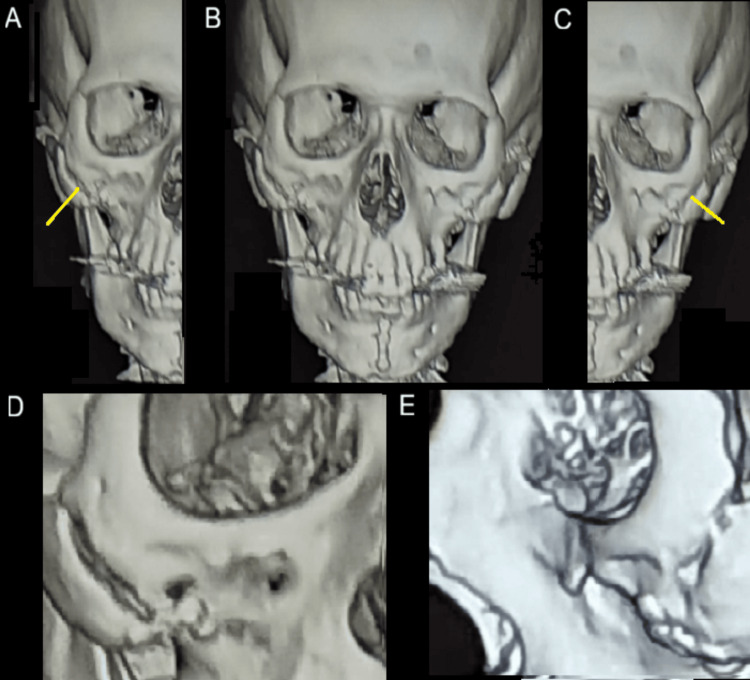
Preoperative radiographic images demonstrating bilateral comminuted zygomatic arch fractures. (A) Right zygomatic arch fracture. (B) Frontal view demonstrating bilateral zygomatic arch fractures. (C) Left zygomatic arch fracture. (D) Close-up view of the right zygomatic arch fracture. (E) Close-up lateral view of the right zygomatic arch fracture.
